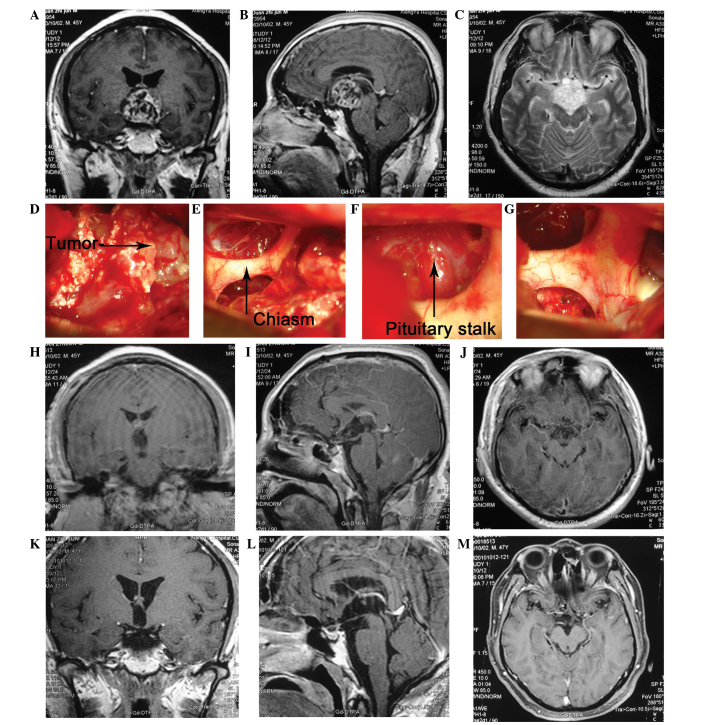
Case of a 45-year-old male who presented with general fatigue for two months, polyuria with blurred vision and memory loss for >1 month. The patient was diagnosed with a CP. During surgery, the tumor was found to originate from the pituitary stalk. In order to completely remove the tumor, the pituitary stalk was also removed. Three years following surgery, the patient resumed work and there was no evidence of recurrence in MRI scans. (A) The coronary view of CE MRI showing that the tumor was located in the sellar and parasellar area. The lesion is mainly solid with contrast enhanced walls. The borders of the tumor are distinct with absence of peritumoral edema. Mild compression of the right side of the third ventricle can also be noted. It also demonstrates the pituitary stalk, the optic nerve and optic chiasm (see arrow). (B) The sagittal view of CE MRI and (C) axial view, shows the relationship between the tumor and the clinoid part of the ICA, optic nerve as well as optic chiasm. (D, E, F and G) The intra-operative findings of the lesion: the tumor was located retrochiasmatically and below the clinoid part of ICA. The pituitary stalk was invaded by the tumor (see arrow). (H, I and J) Post operative CE MRI depicts complete excision of tumor together with absence of hemorrhage. (K, L and M) There was no evidence for tumor recurrence in the 1 year follow up. CP, craniopharyngioma; MRI, magnetic resonance imaging.
